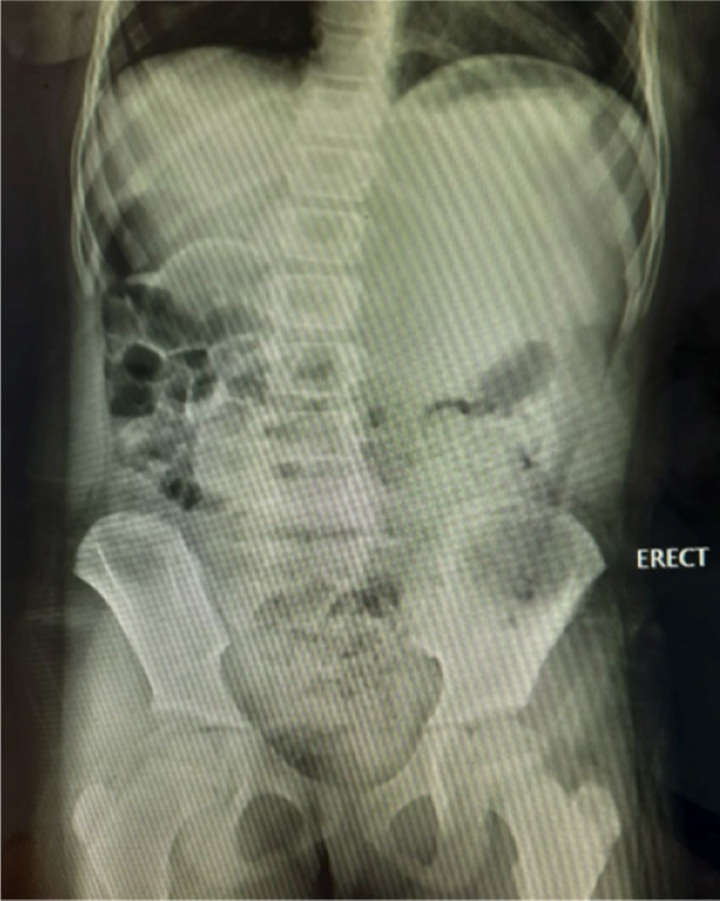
Erect Radiograph of the abdomen showing no intraperitoneal free air.
